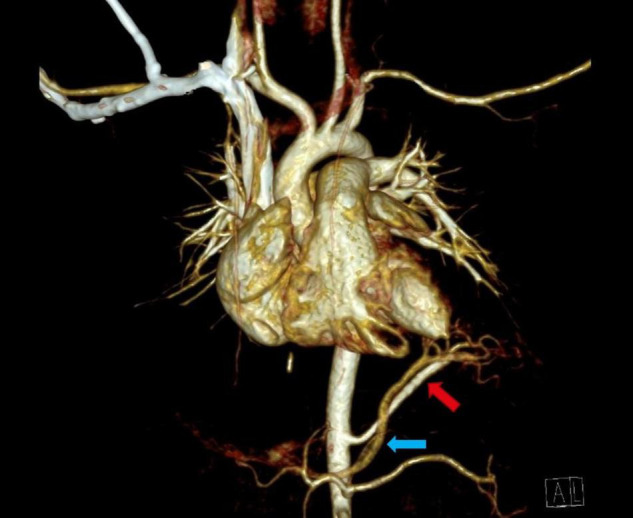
Thoracic contrast-enhanced computed tomography scan (CECT) showing infra-diaphragmatic arterial and venous systemic connection of left inferior extralobar bronchopulmonary sequestration: arterial supply originates from the abdominal aorta (red arrow), venous drainage via portal vein (blue arrow).
